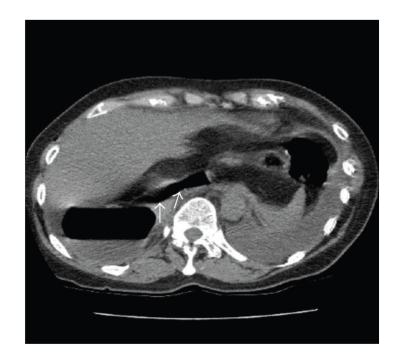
Computerised Tomography (CT) scan herniation of the stomach (Arrows-gastric air within the stomach) into a congenital post-diaphragmatic space.
